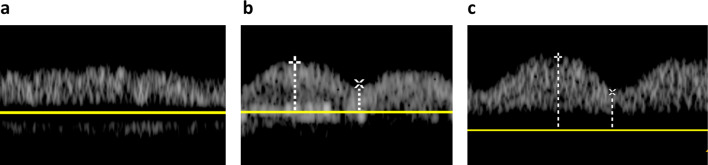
a A normal PV Doppler waveform demonstrates minimal pulsatility, with a pulsatility index < 30%. b A pulsatility index of 30–49% is considered mildly abnormal, c while a pulsatility index > 50% is classified as severely abnormal; PV = Portal Vein
